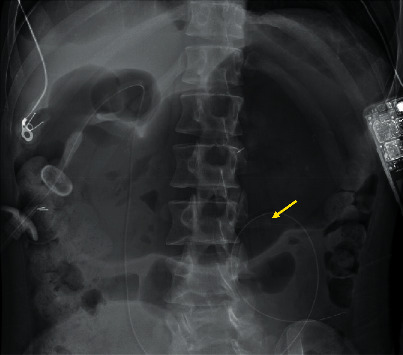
Abdominal X-ray demonstrating severe gaseous distention of the entire stomach with the balloon of presumed gastrostomy projecting over the right abdomen. The tip of a jejunostomy tube placed through the gastrostomy projects over the left midabdomen (yellow arrow). There is no gross evidence of free air noted given inherent limitations of this portable supine radiograph.
